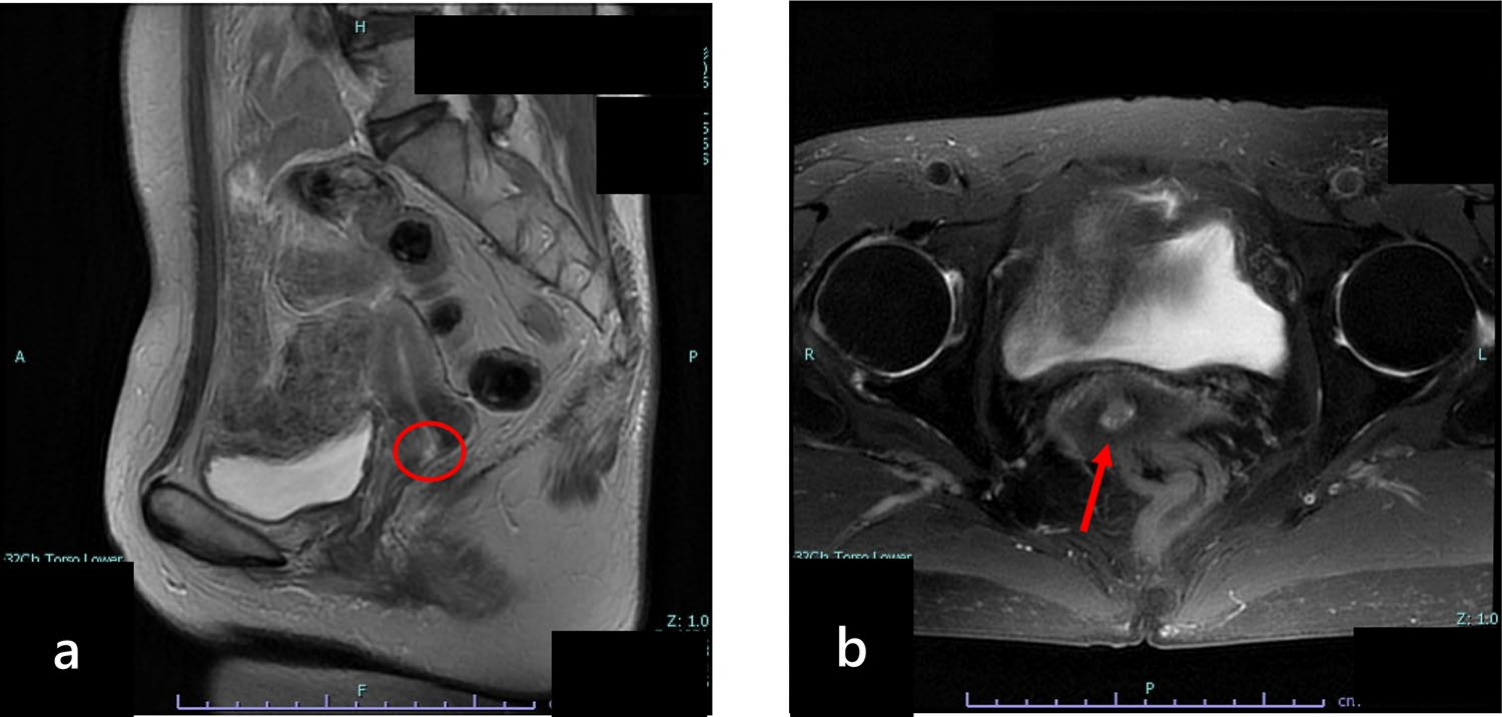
MRI of the pelvis. A faint high-signal intensity area measuring 7 mm in size is visible in the anterior wall of the cervix on T2-weighted MRI. MRI: magnetic resonance imaging. (a, longitudinal image; b, cross-sectional image)
